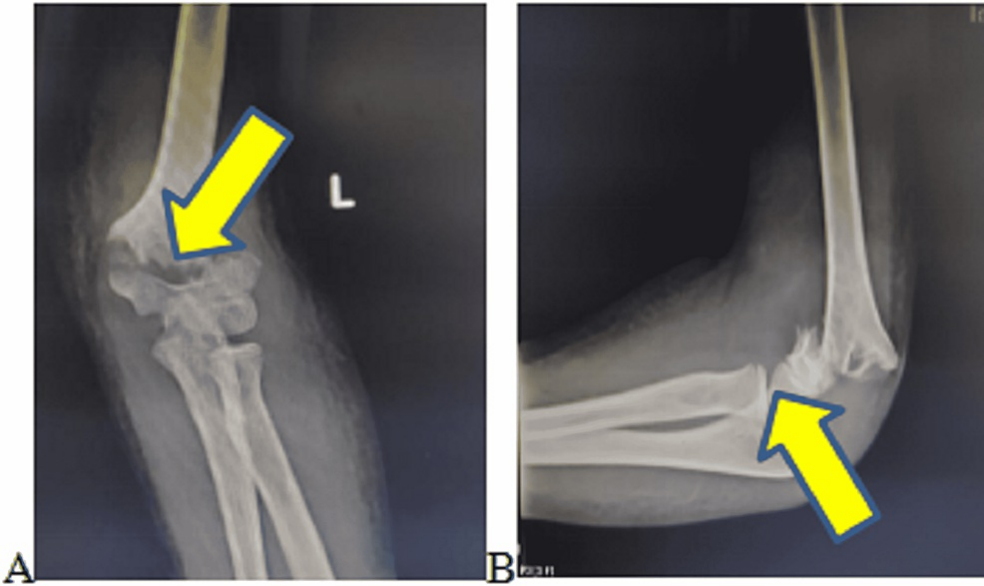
Plain radiograph of left elbow (A) antero-posterior view and (B) lateral view showing left supracondylar fracture with gross rotational anterolateral displacement
